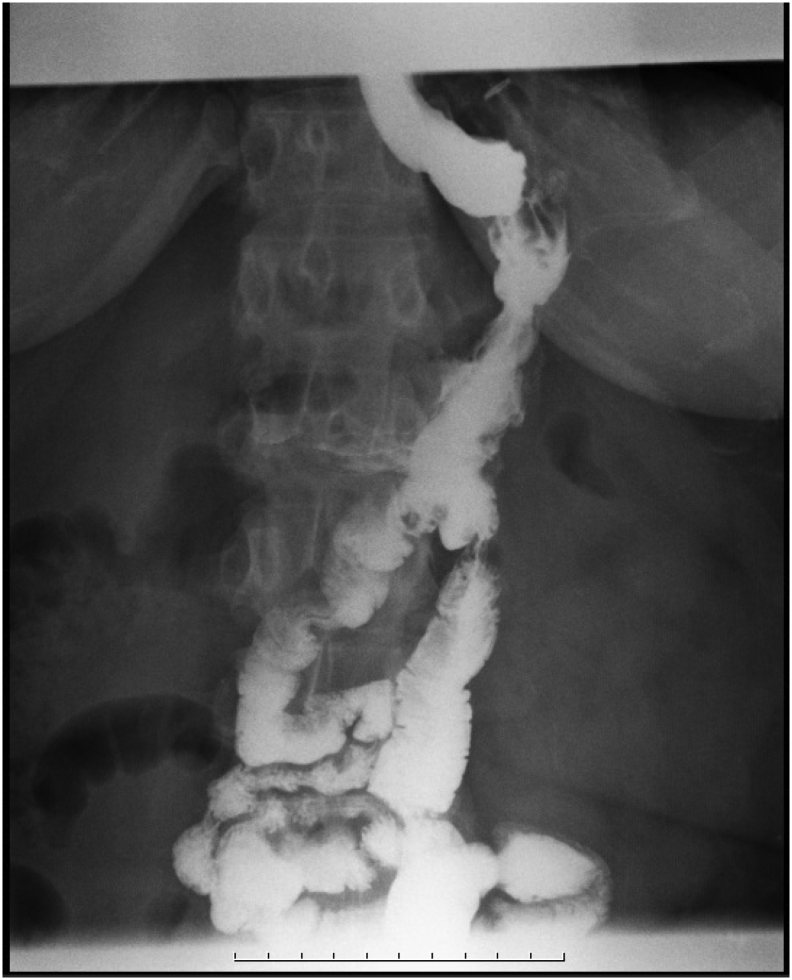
Barium swallow and meal radiography.
